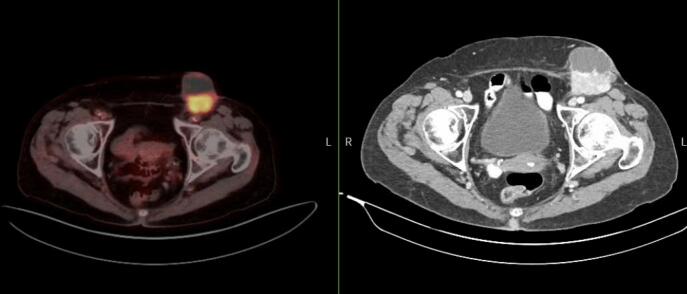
(left) PET-CT scan, Left inguinal nodal mass with solid portion significantly FDG avid consistent with neoplasm. No additional abnormal uptake in the abdomen/pelvis. (right) CT Abdomen and Pelvis, Large, solid, and cystic left inguinal nodal mass with extension into the underlying abdominal wall musculature. This measures about 5.6 × 5.3 cm (previously 4.8 ×  4.6 cm).
